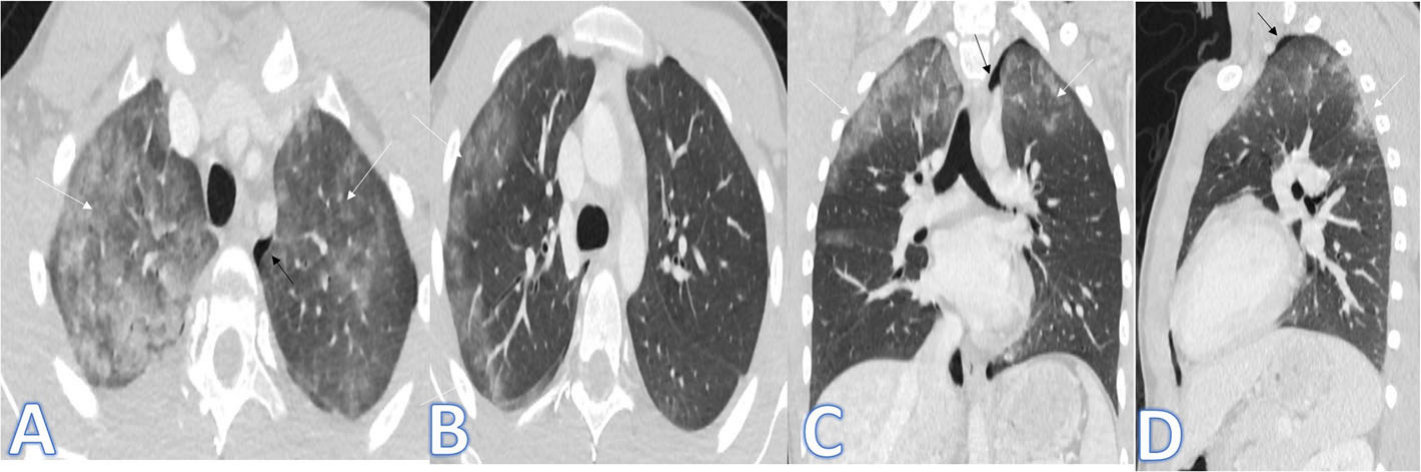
A 19-year-old male patient presented with chest tightness after trauma. He had a history of trauma 1 day ago. CT chest showed bilateral GGO at both the upper lobes and right lower lobes with peripheral predilection (white arrows), the thin rim of the left pneumothorax (black arrow) was seen on the left side, and the diagnosis was post-traumatic pulmonary contusions
